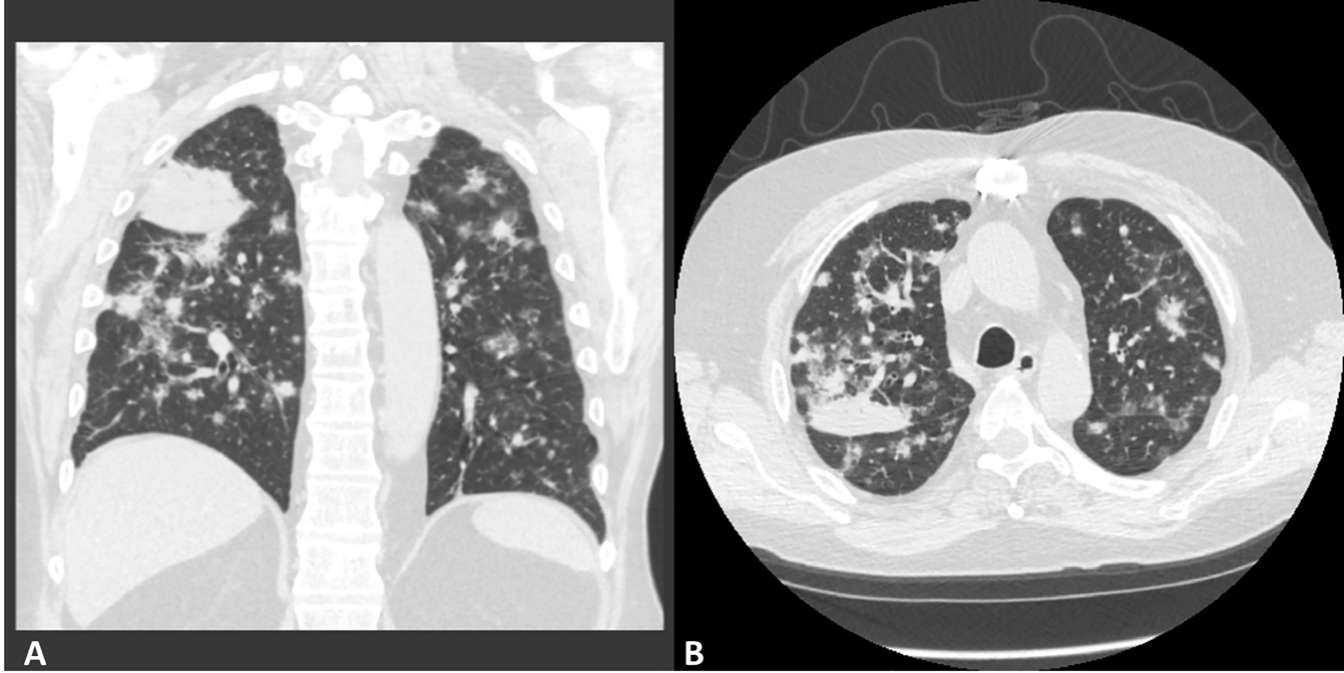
Coronal (A) and axial (B) representative images from the patient’s pre-treatment CT scan.
